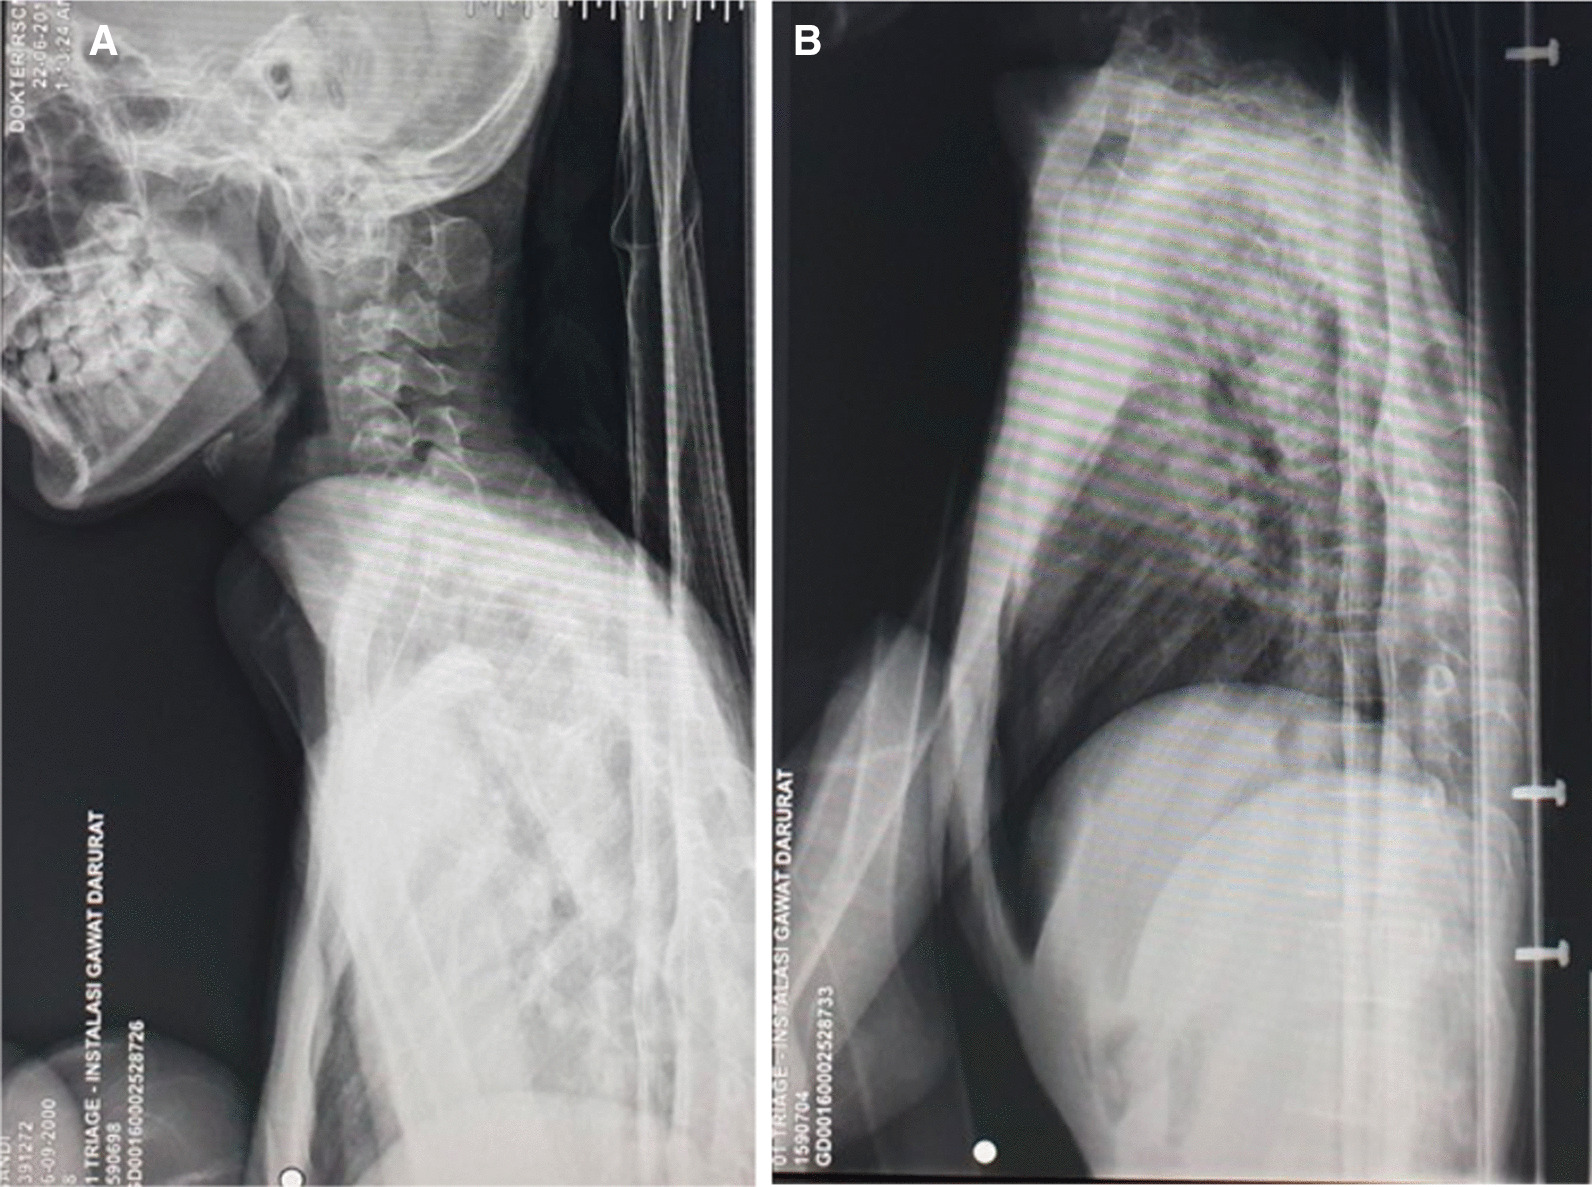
Lateral plain radiographs of cervical spine (A) and thorax (B), showing decreased density and porotic bone without fracture or bone destruction
